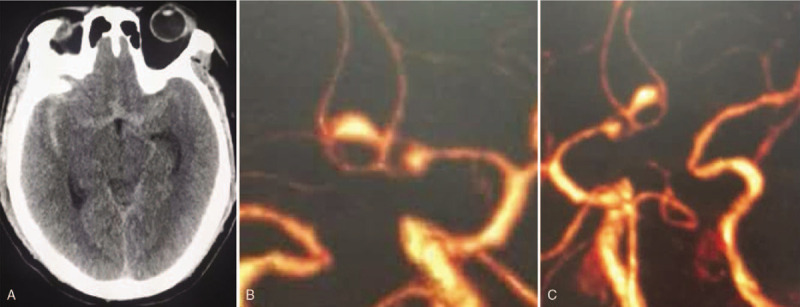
Preoperative head CTA examination. (A) The high-density shadow of the bilateral lateral fissure and the circle of Willis can be seen in the CT axial position, and the ventricle is not significantly enlarged. (B), (C) The anterior communicating artery complex fenestration before revascularization; 2 tandem aneurysms were located at the fenestration, and the right A1 artery is not visible. CTA = computed tomography angiography.
